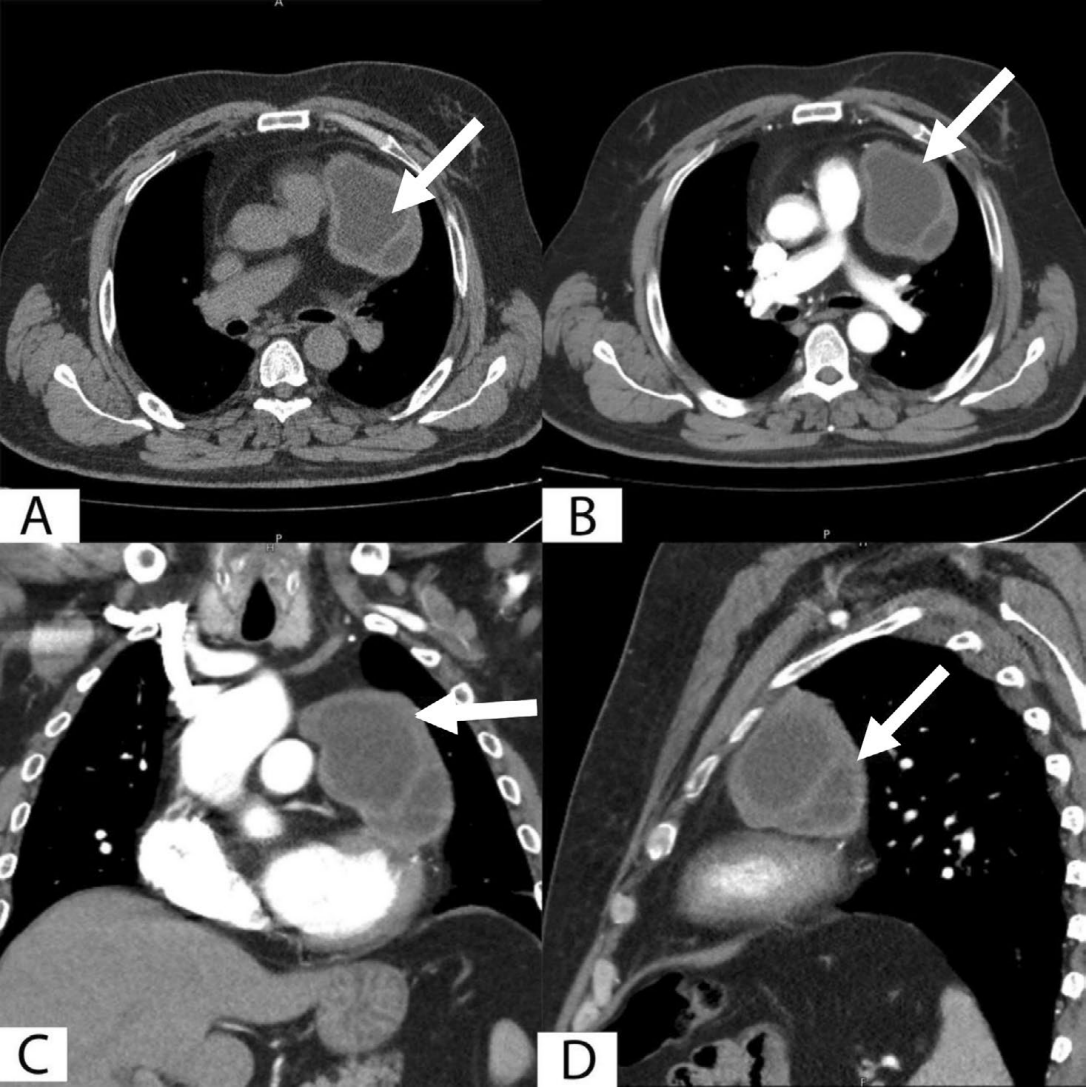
Axial chest CT without iodinated contrast (A) and Multiplanar reconstruction of chest CT imaging with iodinated contrast (B–D) showing a well‐defined mutilocular fluid attenuating nonenhanced lesion (white arrow), located in the left pericardium at the level of the main pulmonary artery, it measures about 8 × 6 cm, most consistent with a hydatid cyst.
